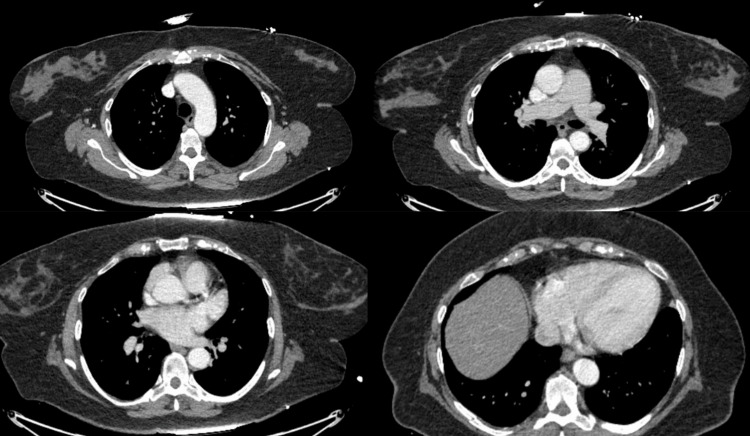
Computed tomography with angiogram (CTA) of the chest on admission CTA of the chest was negative for any acute findings like pulmonary embolism or aortic dissection.
